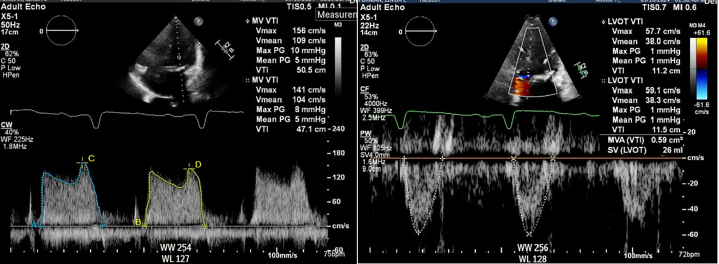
Transthoracic Echocardiography Showing Mitral Valve Area of 0.59 cm^2^ by Velocity Time Integral
